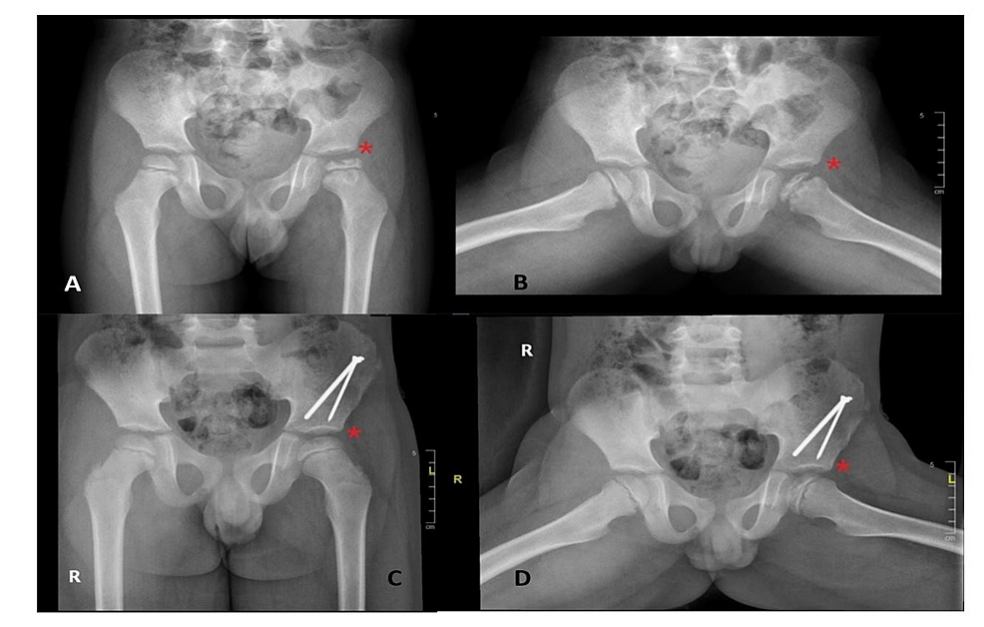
Example of pelvic x-rays of one patient with Legg-Calvé-Perthes disease older than eight years before (A,B) and after (C,D) Non-surgical treatment
